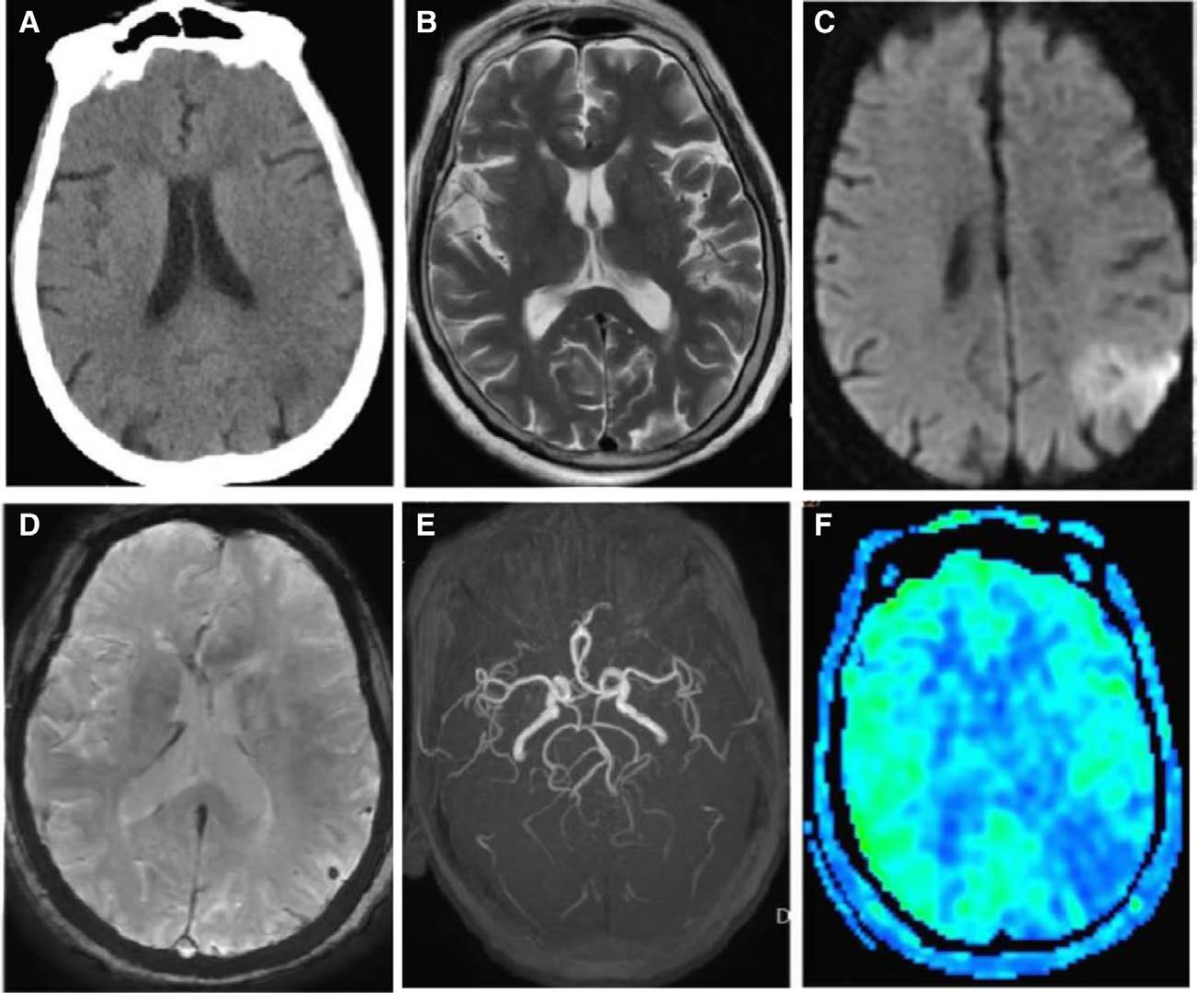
Patient’s imaging examination results. (A) Head CT showing lacunar cerebral infarction in the bilateral basal ganglia region. (B) Brain MRI showing cerebral infarction in the left parieto-occipital region. (C) Brain DWI showing high signal due to diffusion restriction in the left parieto-occipital region. (D) Brain SWAN showing microhemorrhage in the left parieto-occipital region. (E) Brain ASL showing reduced perfusion in the left temporal, parietal, and occipital lobes compared to the contralateral side. (F) Brain MRA showing a slightly narrow lumen of the right anterior cerebral artery. ASL = arterial spin labeling, CT = computed tomography, DWI = diffusion weighted imaging, MRI = magnetic resonance imaging.
